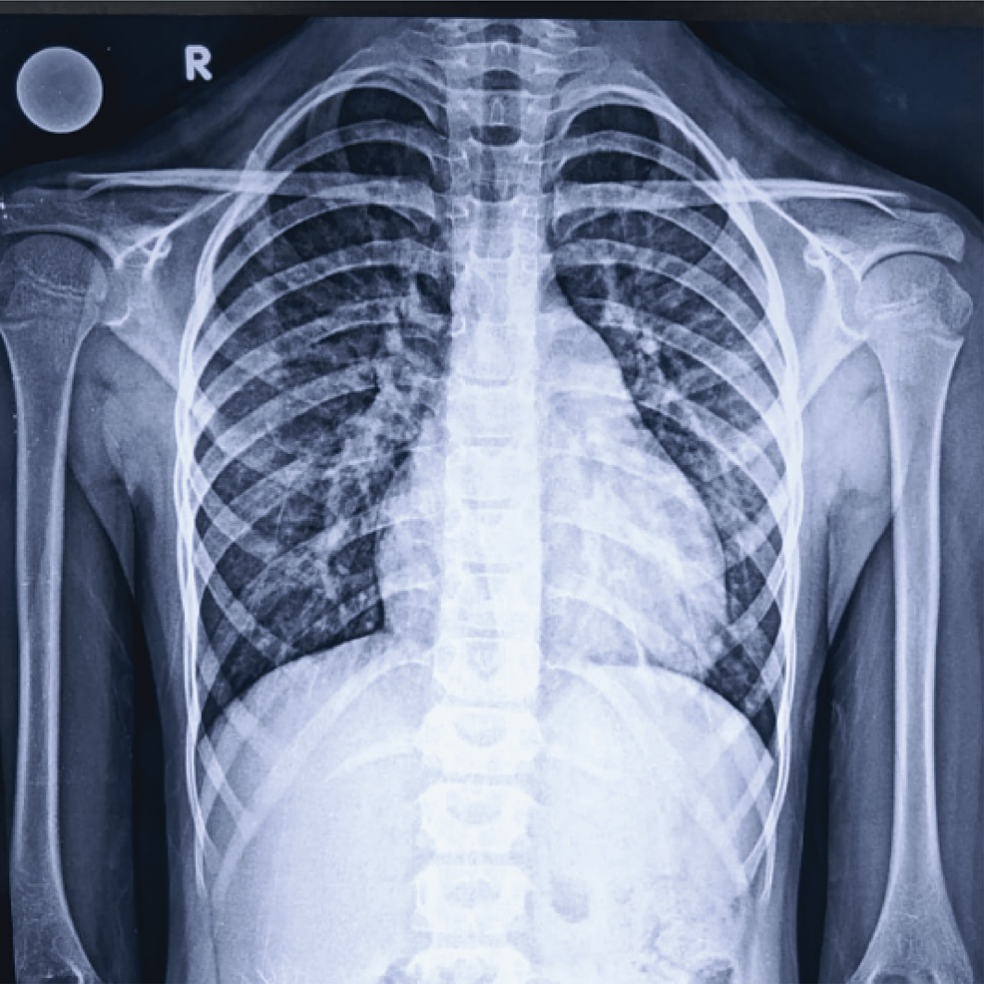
Chest radiograph revealing no abnormality
